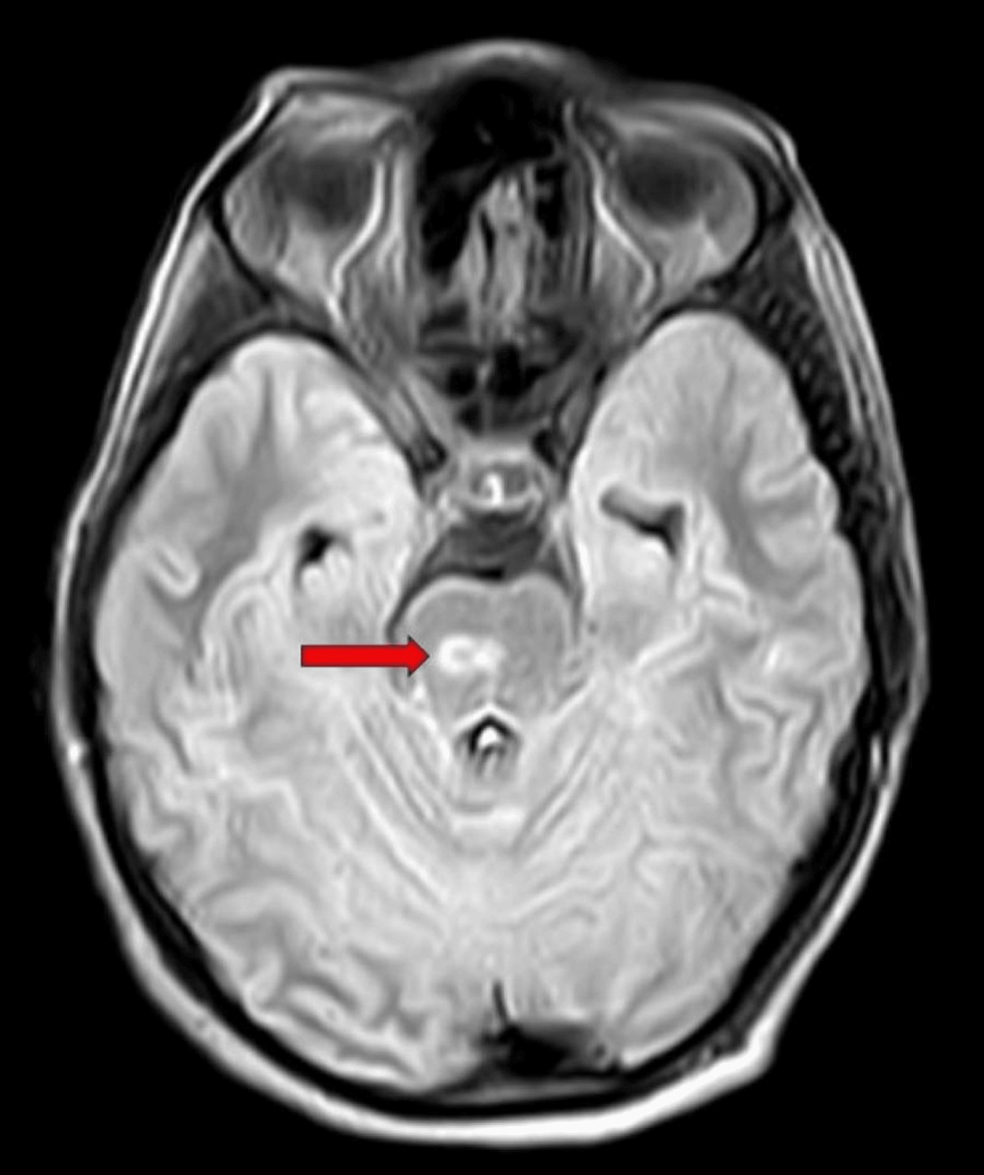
An axial section of magnetic resonance imaging showing a peripherally enhancing altered signal intensity lesion in the pons on the right side (red arrow).
